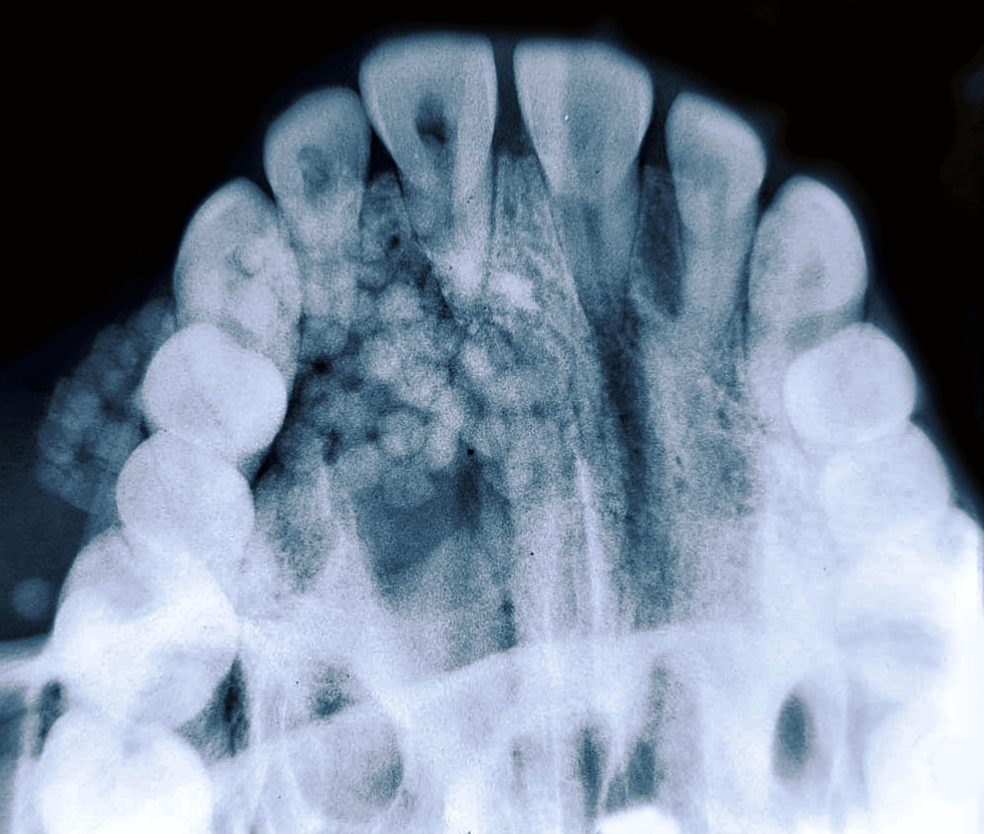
Postoperative occlusal radiograph after seven days. Image Credit: Prasanna Sonar.
